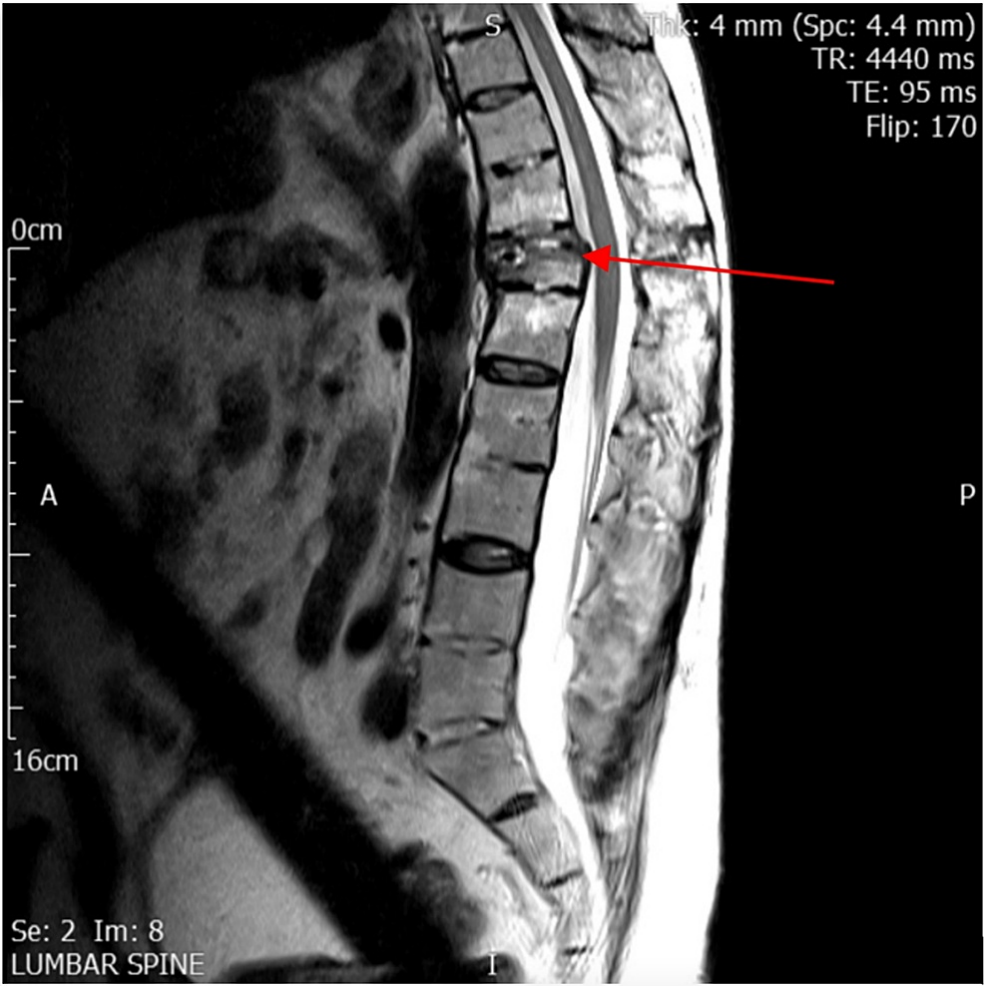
Sagittal T2 MRI image demonstrating T12 fracture non-union (red arrow), note that the image also demonstrates evidence of endplate changes.
